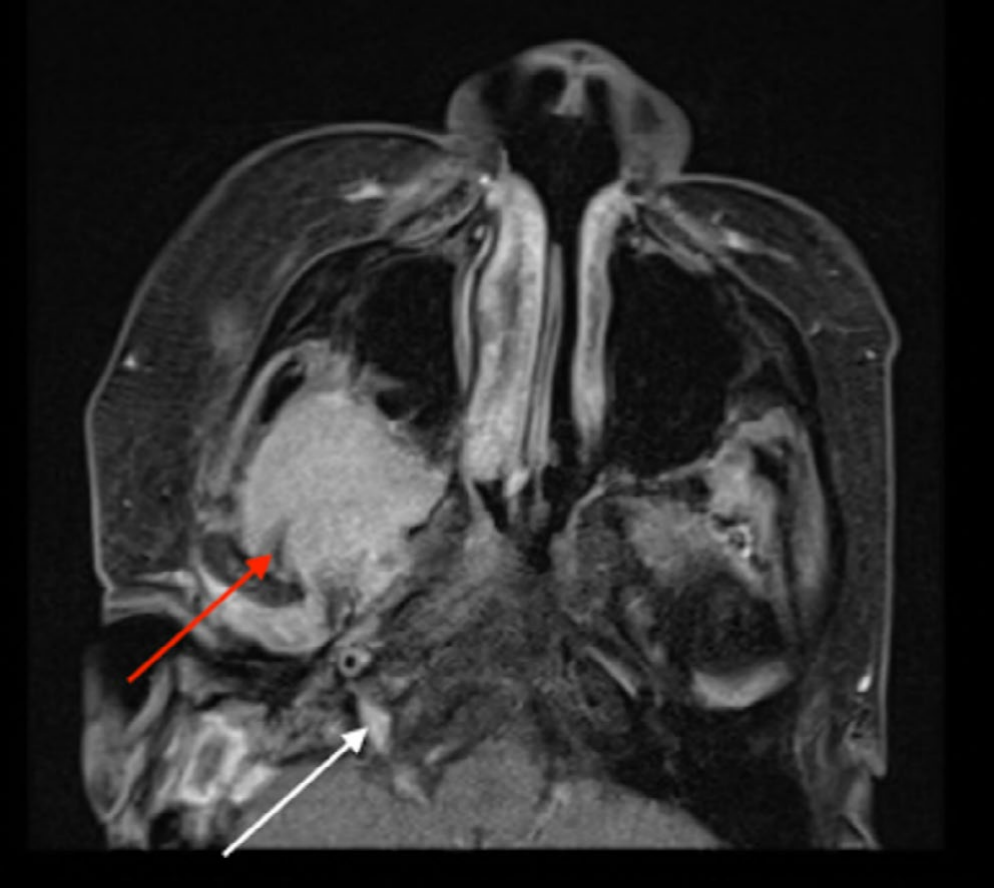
Two‐month postoperative MRI with red arrow indicating recurrence advancing into the right infratemporal fossa (red arrow, changed from previous imaging) with extension through the right hypoglossal canal in close proximity to the right Meckel's cave (white arrow).
